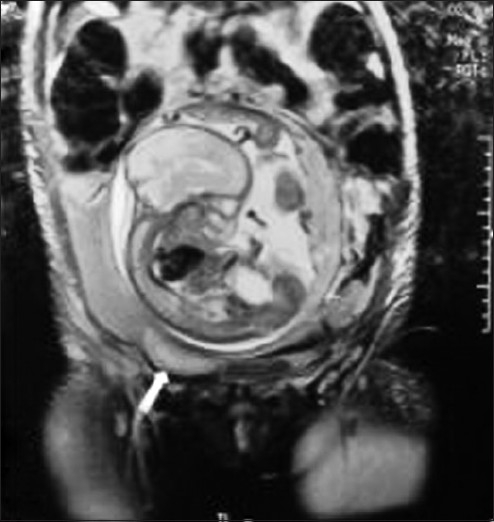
MRI picture showing right uterine horn (arrow) and rudimentary horn above with fetus *in situ*
